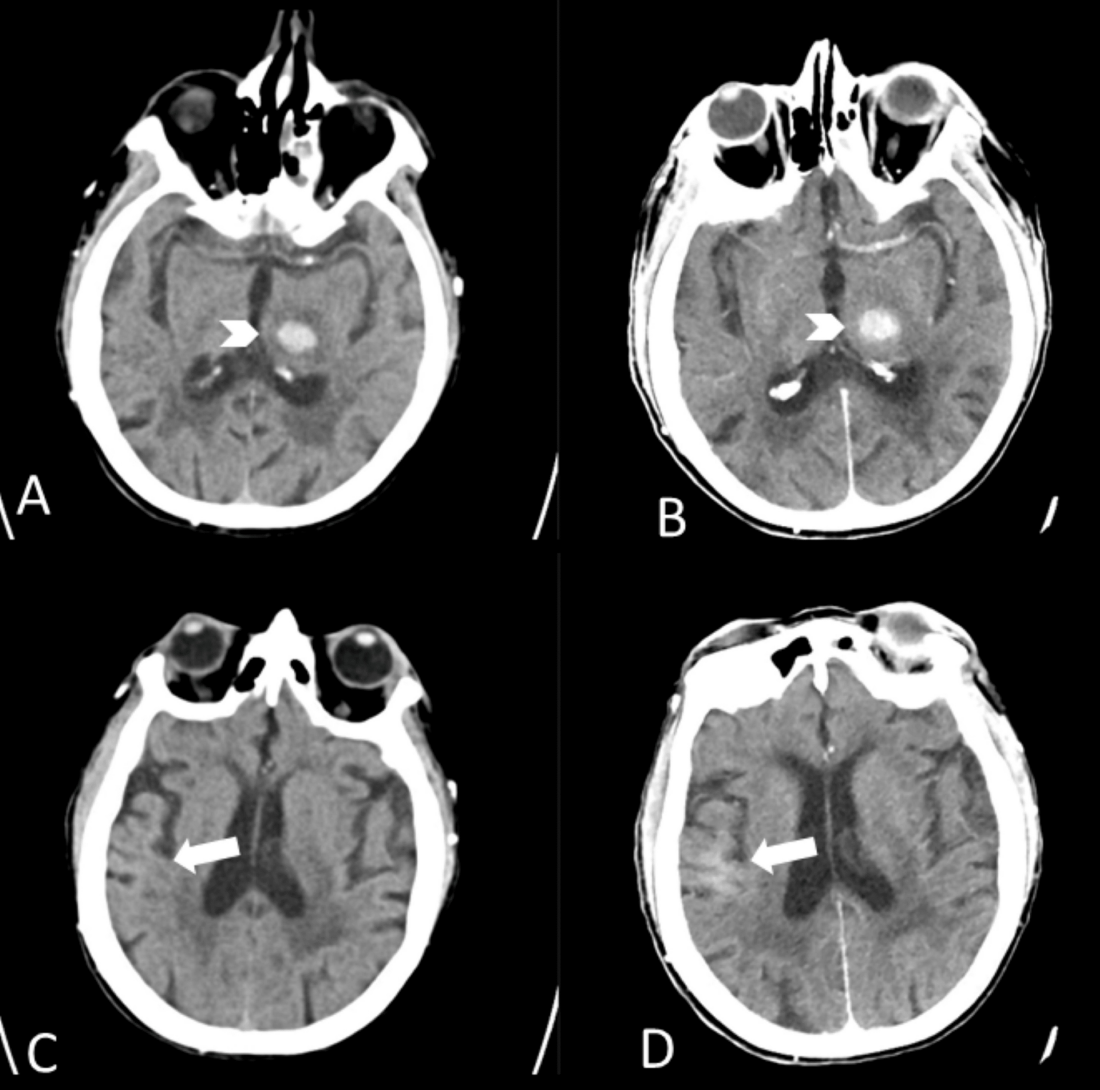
CT images at admission and follow-up non-contrast head CT obtained six hours after CTA of the head and neck A, C: initial CT shows an isolated left thalamic hemorrhage (arrowhead) without any hyperdensity in the right temporoparietal region (arrow); B, D: follow-up CT demonstrates a stable left thalamic hemorrhage (arrowhead) and a new diffuse hyperdensity (arrow) in the right temporoparietal lobe, located superior to the right insula.
